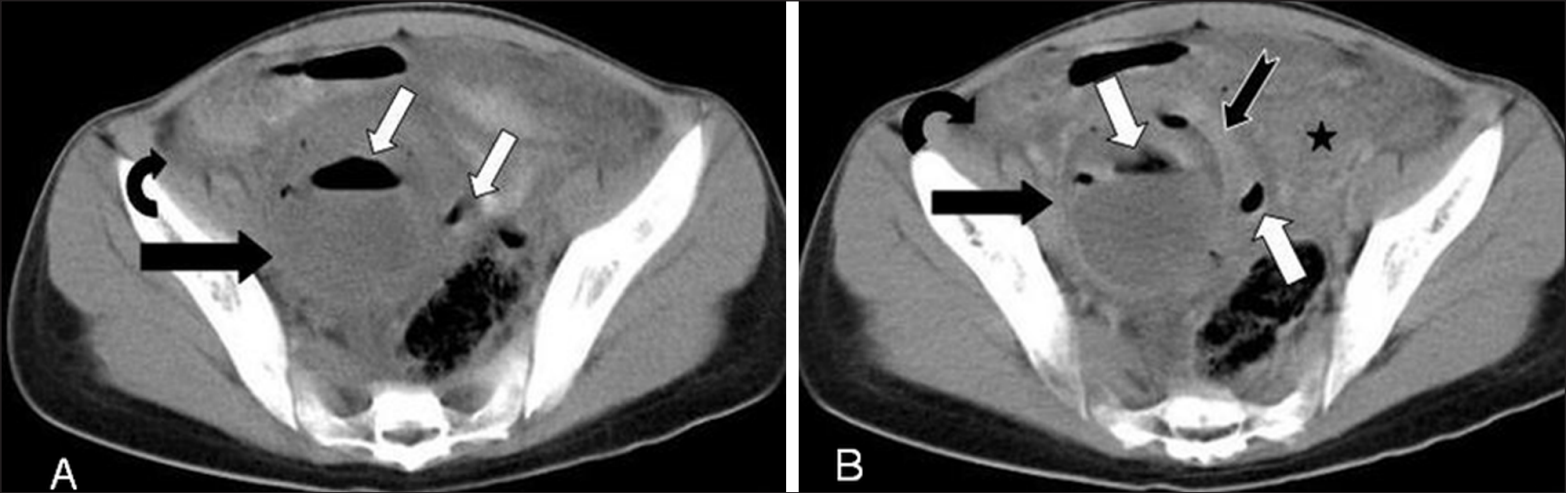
A 14-year-old patient with abdominal pain in right-lower quadrant, who had a history of appendectomy was examined by abdominal CT. Non-contrast (A) and contrast enhanced CT (B) revealed a 7 cm mass (black arrow) in the right adnexal region with air densities (white arrow) and a peripheral rim-type and contrast-uptake of the wall (black jagged arrow), consistent with tubo-ovarian abscess. In the pelvic region, free fluid (curved arrow) around the bowel loops and heterogeneity (star) in the surrounding fat tissue were also observed. The post-operative diagnosis of the case was right tubo-ovarian abscess.
